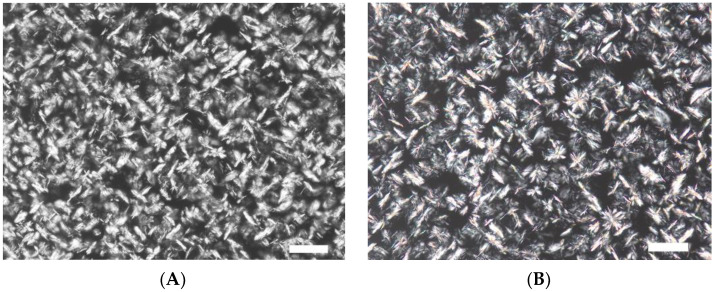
Microstructure of wax oleogels. The control (A) is an oleogel sample obtained without ultrasonic treatment. (B)—an oleogel sample obtained with ultrasonic treatment (Scale 100 µm).
